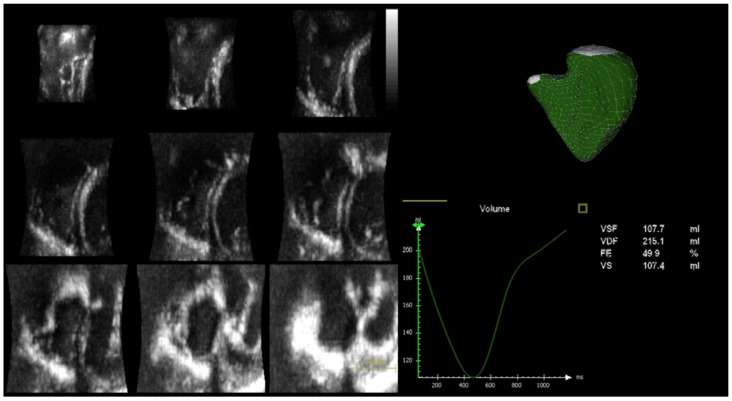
On the left panel, RV full volume 3D 9-slice; on the right panel, determination of end-diastolic and end-systolic volumes (EDV and ESV) and RV ejection fraction (RV EF) by 3D echocardiographic analysis.
